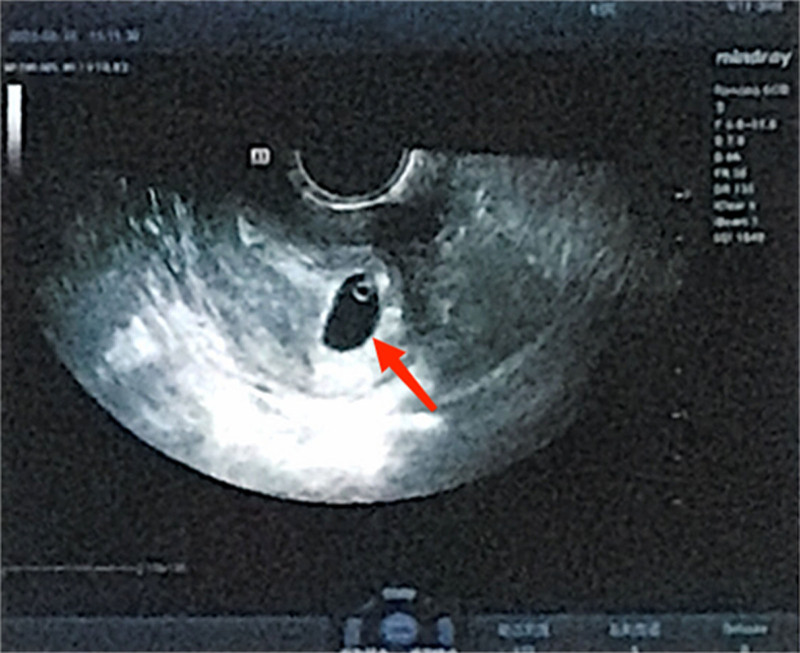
(August 2023) Transvaginal ultrasound showed a pulsating fetal heartbeat, revealed an intrauterine pregnancy with a live fetus at approximately 6 weeks gestation.
